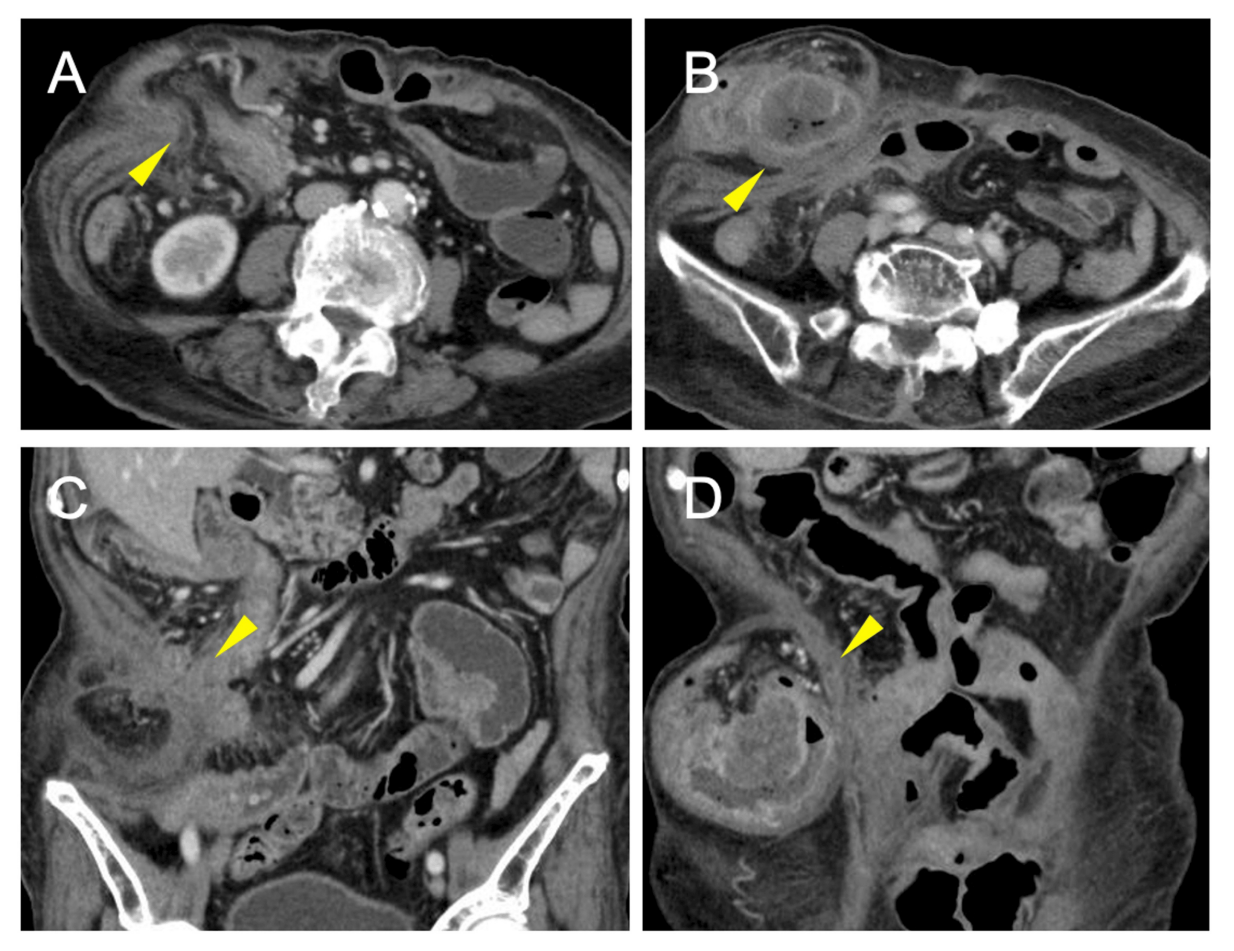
Contrast-enhanced CT. (A and B) Axial images showing an incisional hernia at the right subcostal region containing the ascending colon with wall thickening and diminished enhancement. (C and D) Coronal images showing the ascending colon herniating through the fascial defect with surrounding fat stranding.
